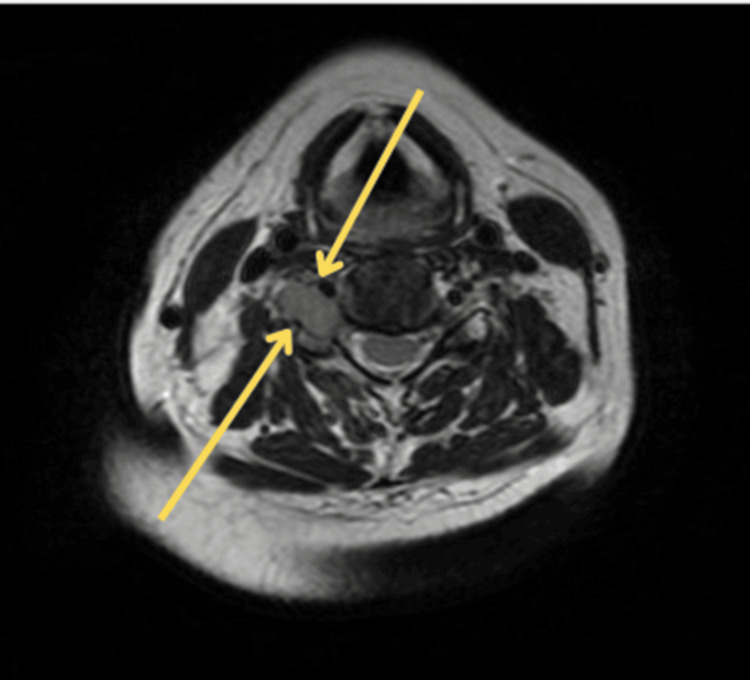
Enhancing right C5 nerve root on MRI Axial MRI of the cervical spine demonstrating a well-defined enhancing lesion involving the right C5 nerve root (yellow arrows), suggestive of lymphomatous infiltration.
